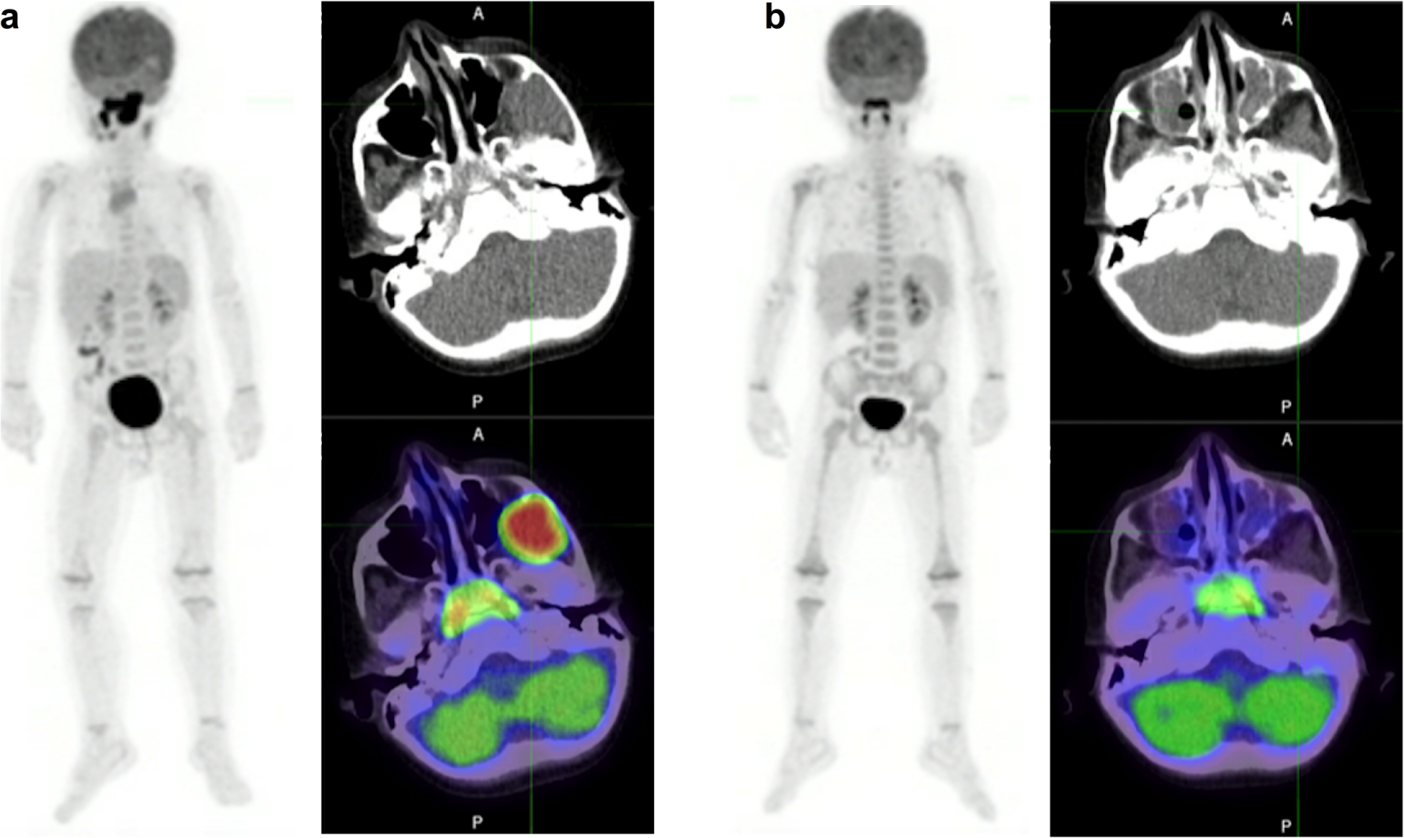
Rhabdomyosarcoma on the left pterygoid fossa in a 6 year-old child treated with chemotherapy demonstrating the marked suppression of cortical brain uptake associated with general anaesthesia (a). Note that the same technique was applied for the therapy response scan (b). From left to right, MIP body PET CT and PET/CT fused axial slices
